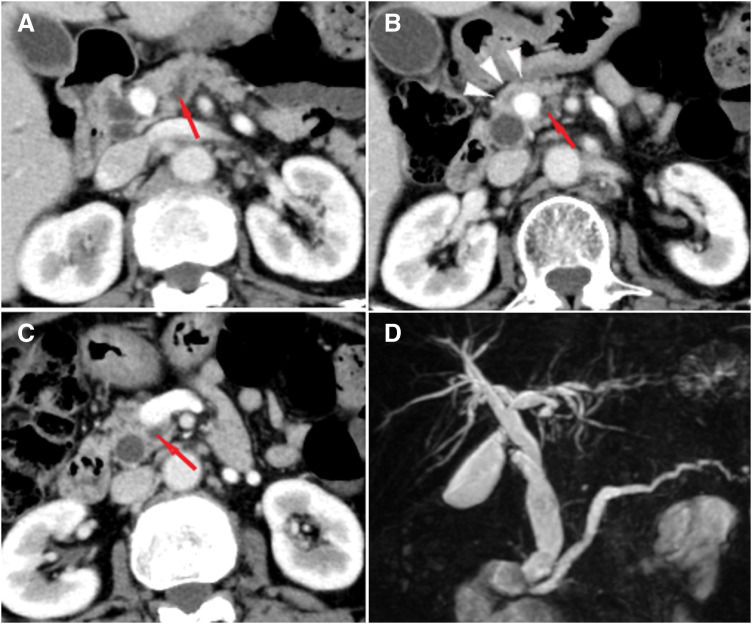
CT and MRCP images. (A) Dilation of the main pancreatic duct observed on CT imaging (red arrow). (B) The portal vein parenchyma is observed on the anterior surface of the pancreas (white triangle), but the dilated main pancreatic duct is located dorsal to the portal vein (red arrow). (C) Slightly dilated main pancreatic duct observed dorsal to the portal vein (red arrow). (D) MRCP shows dilatation of the main pancreatic duct with no obvious abnormality. MRCP, magnetic resonance cholangiopancreatography
